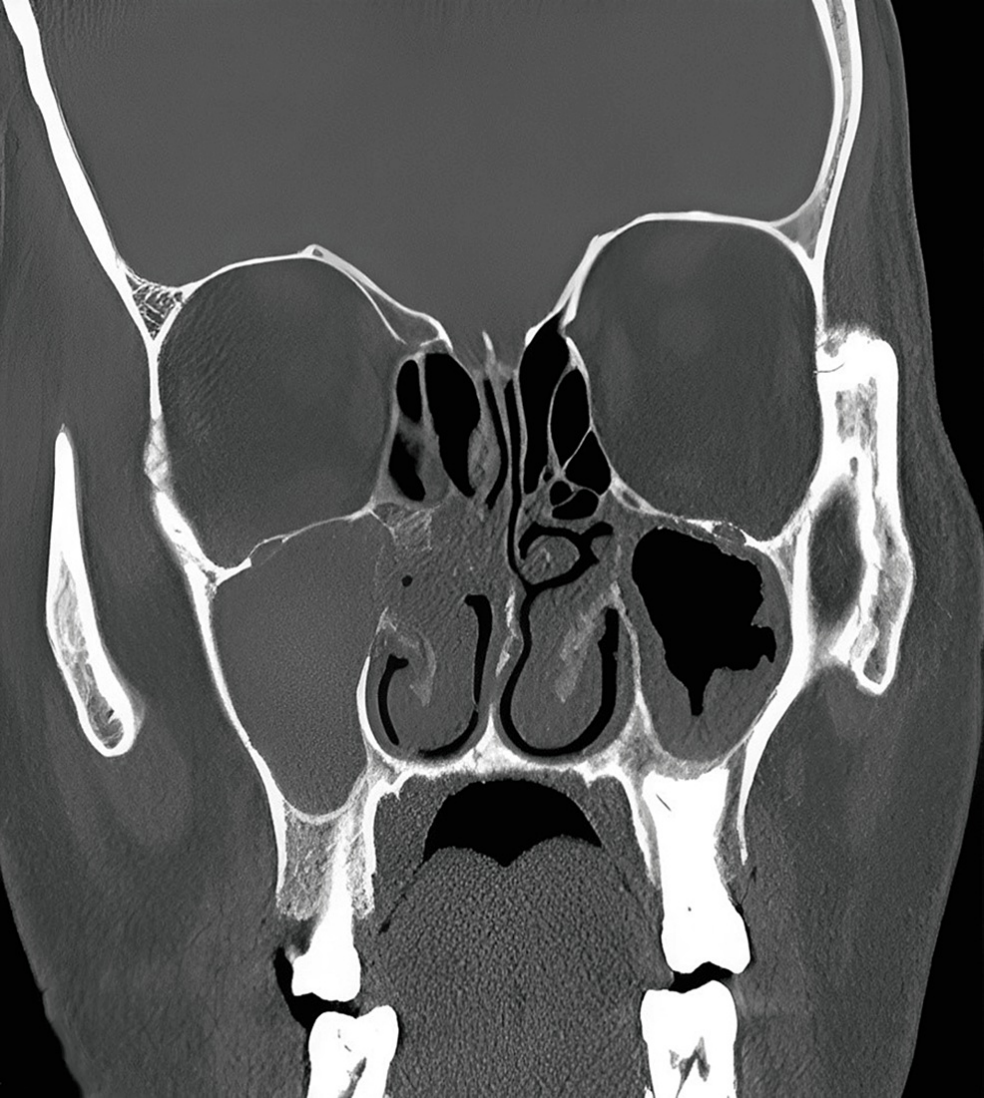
CT scan of the paranasal sinuses. Opacification of the maxillary sinus, ethmoid sinus, middle meatus, and turbinates is evident and more pronounced on the right side. CT: Computed tomography
